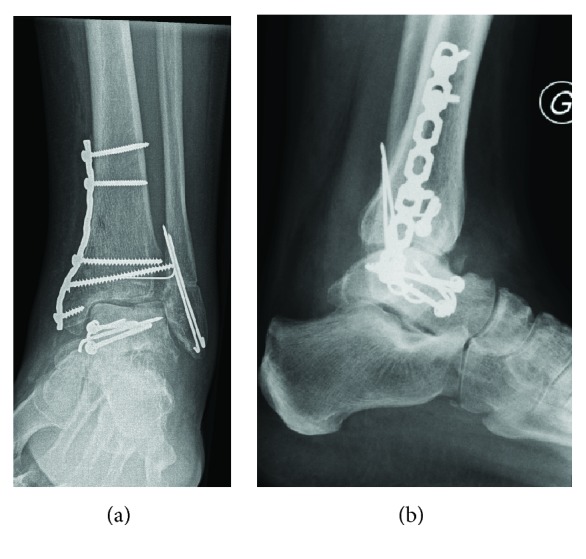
(a) AP and (b) lateral X-rays of the left ankle at 6 months, showing consolidated fractures and an anatomical reconstruction, with the absence of necrosis of the talus.
